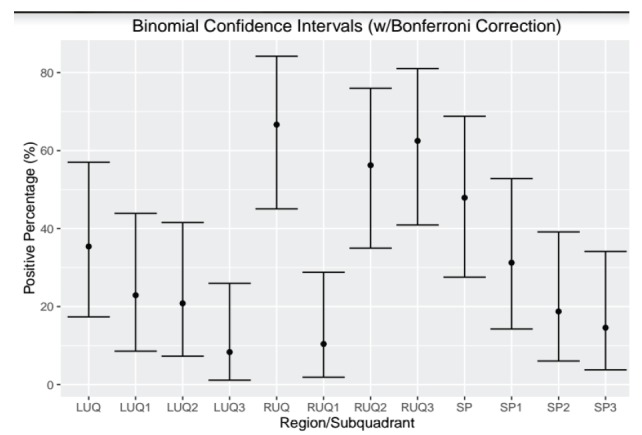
This plot gives the percentage of positive results from each sub-quadrant and quadrant with 95% confidence intervals around those estimates using the method of Clopper and Pearson with a Bonferroni correction to account for the multiple comparisons. As noted, the right upper quadrant (RUQ) is the most positive quadrant, and the caudal edge of the liver (RUQ3) is the most positive sub-quadrant among all reported FAST exams. *LUQ,* left upper quadrant; *LUQ1,* spleno-diaphragmatic space; *LUQ2,* spleno-renal space; *LUQ3*, inferior pole of the left kidney; *RUQ*, right upper quadrant; *RUQ1*, hepato-diaphragmatic space; *RUQ2*, hepato-renal space; *RUQ3,* caudal edge of the liver; *SP*, Supra-pubic; *SP1,* lateral on either or both sides of bladder; *SP2*, posterior to bladder and anterior pelvic organs; *SP3,* posterior to uterus, or pelvic cul-de-sac; *FAST*, focused assessment with sonography in trauma exams.
